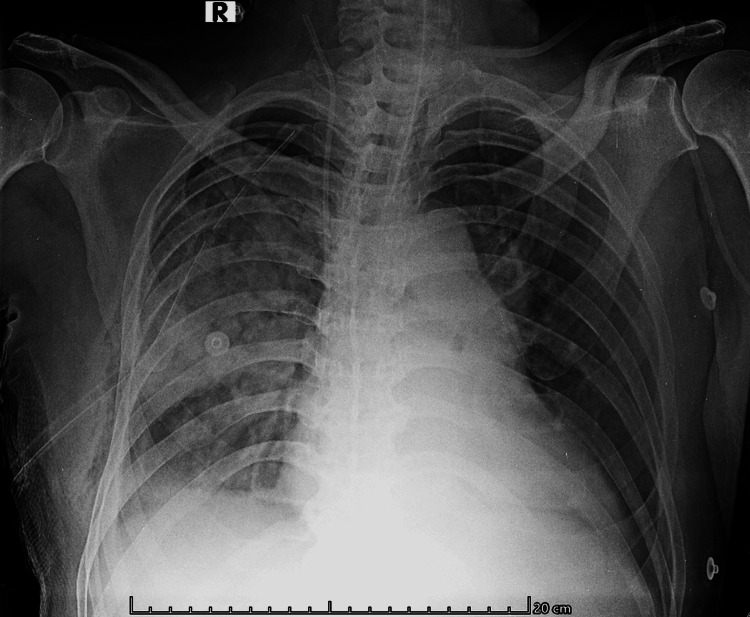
Post–intercostal chest drain portable chest radiograph (anteroposterior view) Portable anteroposterior (AP) chest radiograph obtained after decompression shows a right intercostal chest drain (ICD) coursing cranially along the lateral right hemithorax with the tip projected over the mid-to-upper right chest. An endotracheal tube (ETT) is in situ with the tip above the carina, and a central venous catheter (CVC) is visible. Persistent right-sided air-space consolidation is present with basal volume loss and blunting of the right costophrenic angle, compatible with a small residual effusion. No definite pleural line or deep sulcus sign is seen, indicating no radiographic evidence of a large residual pneumothorax.
